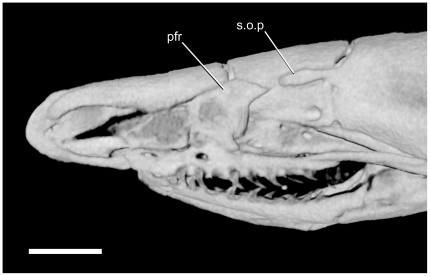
Lack of contact between prefrontal and supraorbital process of parietal of *Uropeltis woodmasoni* (TMM M-10006). Reconstruction from CT scan, left lateral view, anterior to the left. pfr = prefrontal; s.o.p = supraorbital process of the parietal.
